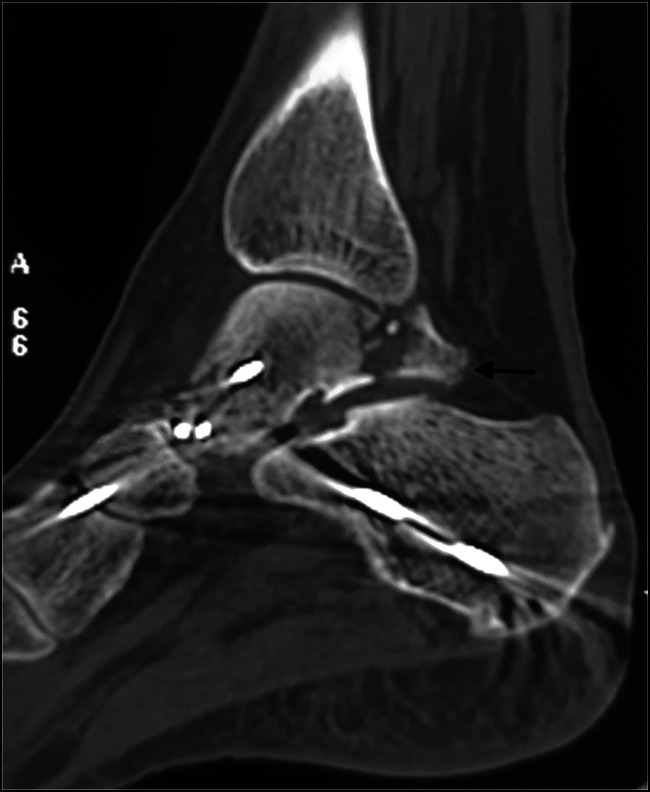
CT scan obtained after closed reduction and percutaneous pinning of a medial subtalar dislocation, which was unstable after reduction. Note the posterior process fracture extending into the subtalar joint. Large fragments such as these need to be fixed.
